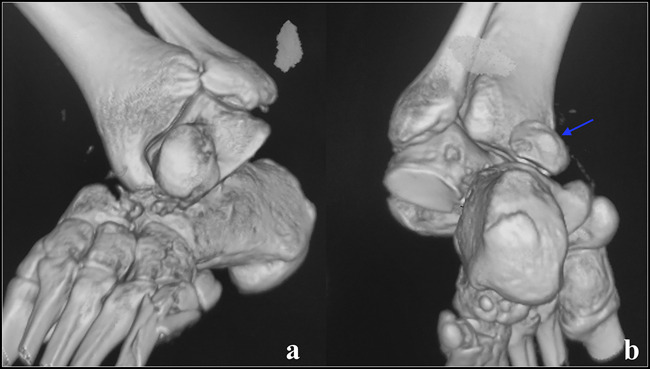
CT scan with volumetric three-dimensional reconstruction of a patient with irreducible medial subtalar dislocation. There is loss of articulation of the talonavicular and subtalar joints. The posterior process fracture is also noted (arrow).
